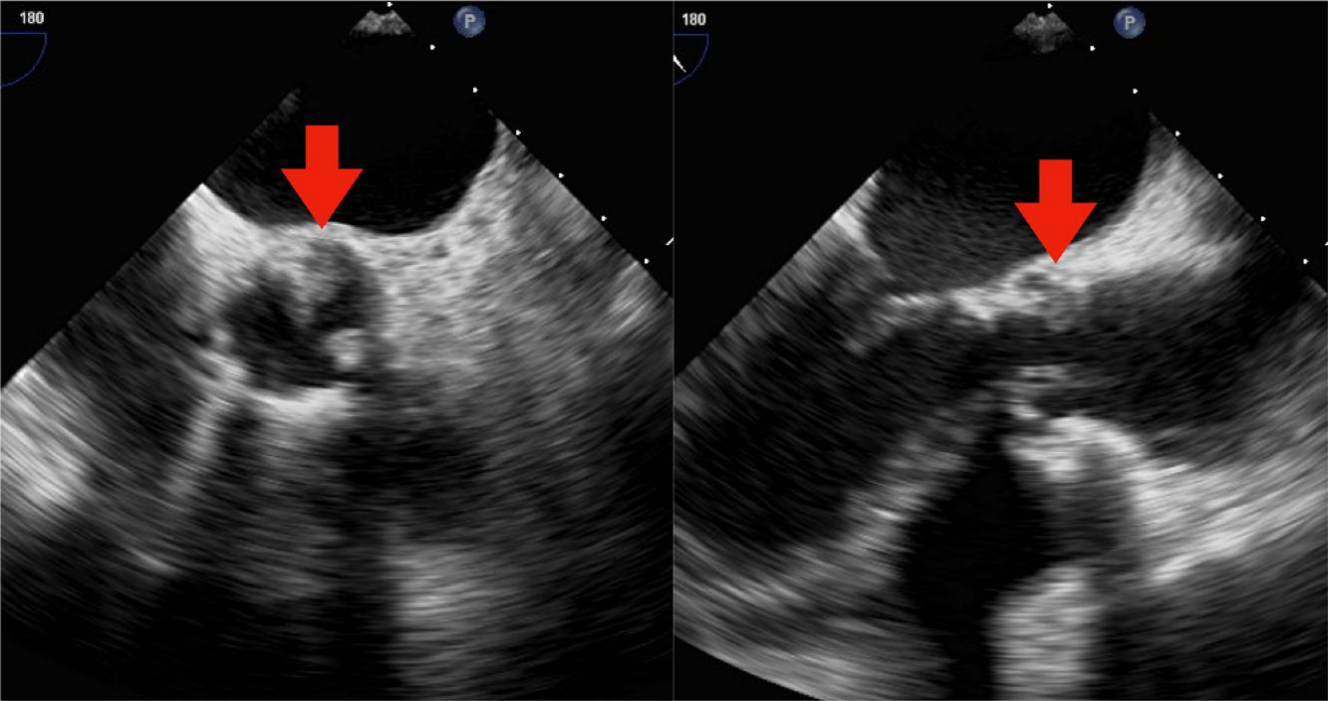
Transoesophageal echocardiography performed in May 2023 showing prosthetic aortic valve vegetation.
